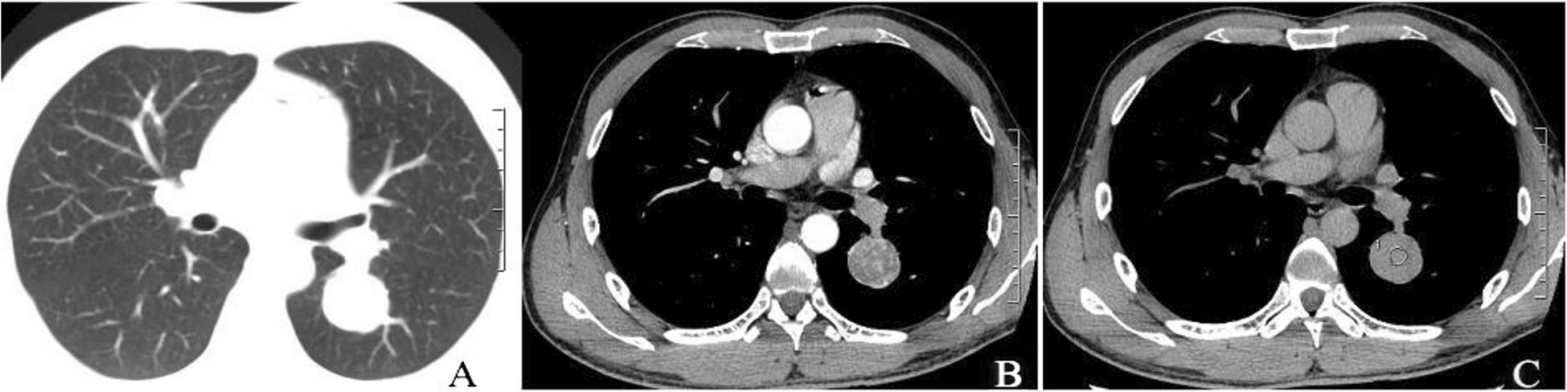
Chest computed tomography findings of the tumor. (A) Chest plain computed tomography scan shows a solitary, rounded, smooth-surfaced, homogenous density mass of approximately 3.4 cm in diameter in the left lower lobe (S6). (B) The contrast-enhanced arterial phase shows intense heterogeneous enhancement measuring 67.2 to 128.0 Hounsfield units. (C) The contrast-enhanced delay phase shows an isodense nodule measuring 66.3 Hounsfield units.
